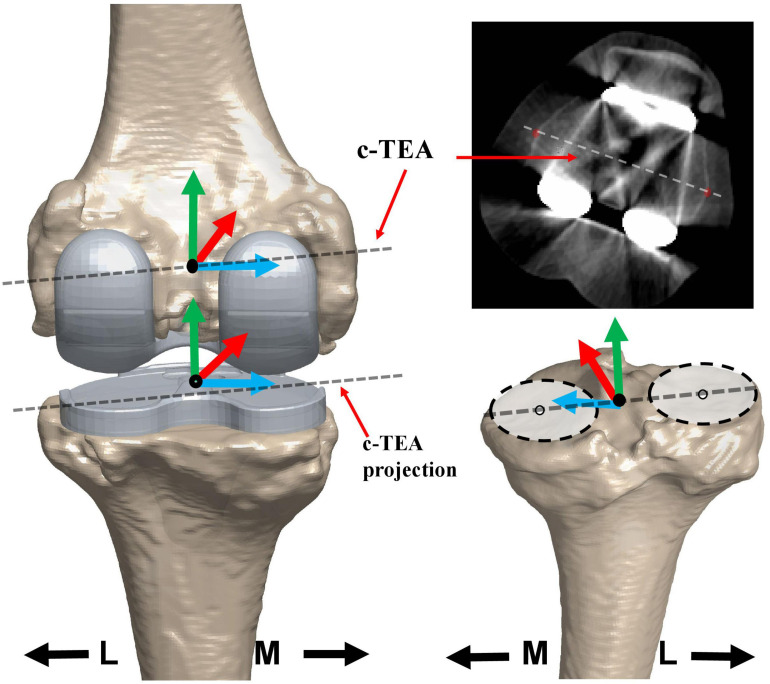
The local coordinate system of the femur and the tibia. The coordinate systems of the femur and tibia are based on the 3D knee model of MP TKA patients reconstructed from computer tomography (CT) images. The dotted line on the 3D model and in the CT image of the femoral prostheses was the clinical trans-epicondylar axis (TEA) of the femur. The dotted line on the tibial prosthesis was the projection of the clinical trans-epicondylar axis (TEA). The dotted line on the non-operated tibial plateau was the line connecting the two best-fitted circles. The black solid point indicated the center of the femur or tibia. The red, green, and blue lines showed the anterior/posterior (AP), proximal/distal (PD), and medial/lateral (ML) axes, respectively.
